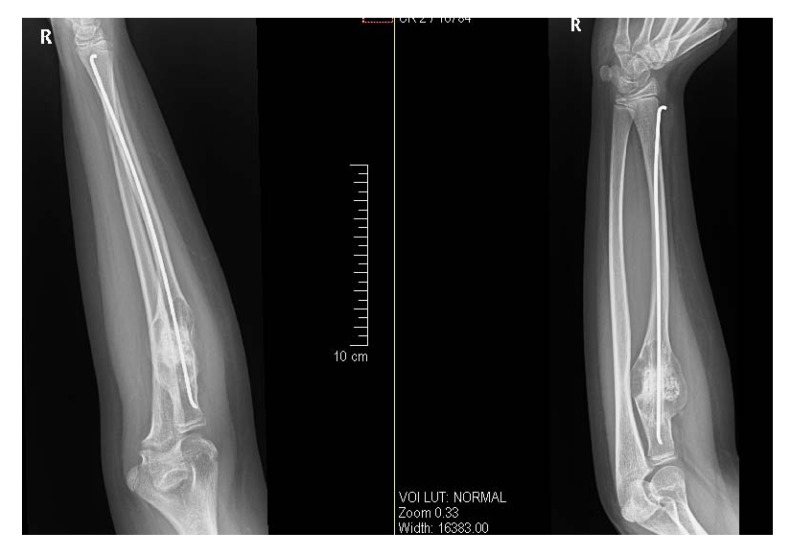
One year follow-up post-operative radiograph showing incorporation of allograft and fibular strut graft with bony union and without any signs of recurrence of tumor.
